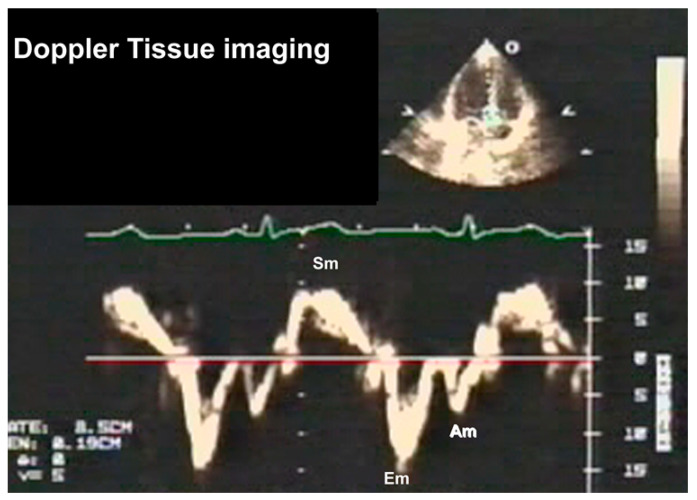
Components of mitral annular velocities obtained by Doppler tissue imaging from the LV apical view. The Sm wave is produced by the mitral annular descent, resulting in this apically directed velocity, the Em or E’ early diastolic velocity, which is produced by mitral annular ascent because of LV relaxation and is a good index of rate of LV relaxation and the Am or A’ velocity because of atrial contraction causing further annular ascent. The E’ velocity varies by the mitral annular quadrant and is generally >8 cm/s for the medial mitral annulus and >10 cm/s for the lateral annulus. Impaired LV relaxation causes a reduction in these velocities. Regional abnormalities will affect these regionally. In constrictive pericarditis, where the lateral LV wall is tethered to the pericardium, the lateral annular E’ velocity will be lower than the medial E’ velocity as the medial annulus compensates for the restricted LV lateral wall long axis function.
